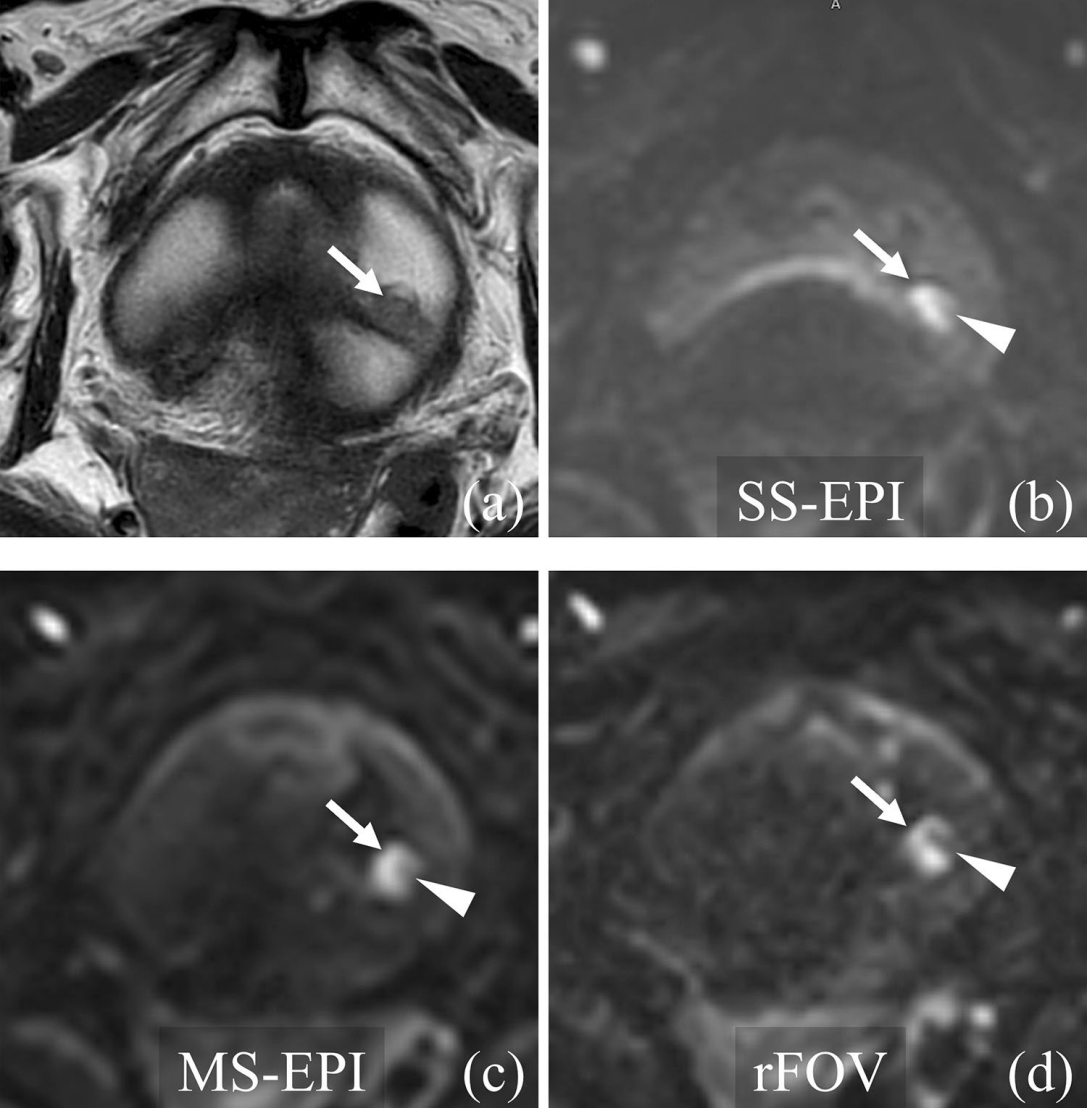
Qualitative evaluation of lesion conspicuity in a 79 year-old man with non-muscle invasive bladder cancer. a T2-weighted image shows a tumor (white arrow) protruding from the posterior bladder wall. b Single-shot echo planar imaging diffusion weighted imaging (SS-EPI DWI). The tumor (white arrow) is clearly recognizable as an area of high signal, but is slightly deformed and the tumor stalk (white arrowhead) is indistinct. c Multi-shot echo planar imaging diffusion weighted imaging (MS-EPI DWI). The tumor (white arrow) is recognizable as an area of high signal with a clear lesion margin and the tumor stalk (white arrowhead) is well defined compared to SS-EPI DWI. d Reduced field-of-view diffusion weighted imaging (rFOV DWI). The tumor (white arrow) is recognizable as an area of high signal with a clear lesion margin and the tumor stalk (white arrowhead) is well defined compared to SS-EPI DWI
